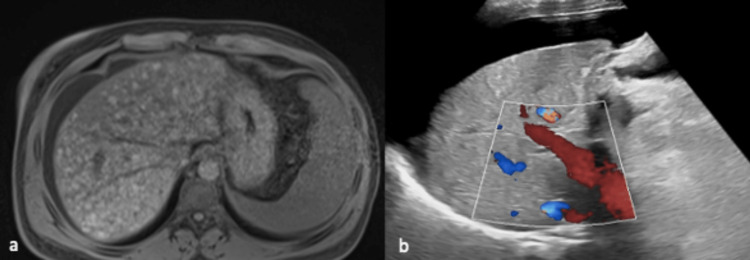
Abdominal MRI and ultrasound a. MRI of abdomen and pelvis T1 with contrast showing cirrhosis with multiple regenerative nodules. b. Abdominal US with Doppler showing patent hepatic vasculature.
